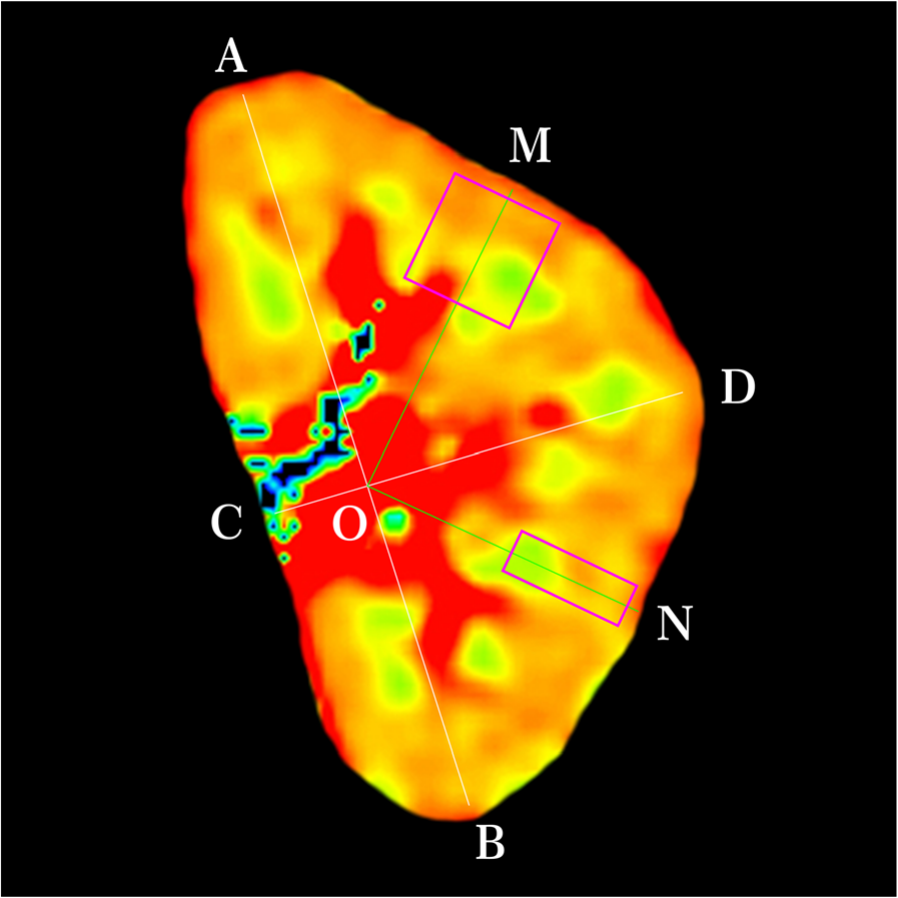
Establishment of ROIs for the renal DWI map. This coronal section of the renal DWI map came from the right kidney of a patient with LN. The line CD linked the renal middle pole and the renal hilus. The line AB was connected between the renal upper pole and the lower pole. Point O was the junction of the two lines. In order to acquire consecutive ADC data along the direction from the superficial cortex to the deep layer of the medulla, the rectangular ROI and squared ROI were placed in the renal-parenchyma BOLD image. One tip of the ROI was located on the renal-cortical surface. Another tip of the ROI was oriented toward the point O. The long axes of the rectangular ROI and squared ROI are shown by the green lines
